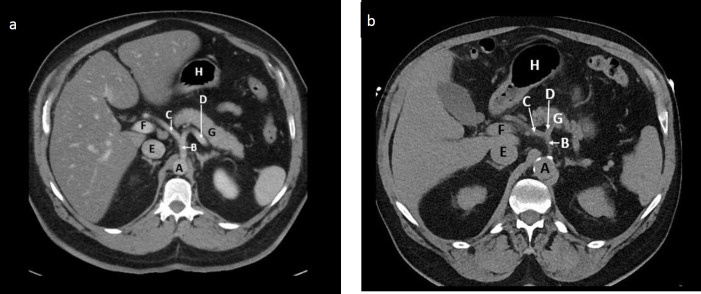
Contrast enhanced (a) and non-contrast (b) axial CT abdomen of two different patients at the level of the coeliac artery origin is shown. Identify the normal anatomical structures labelled A to H
